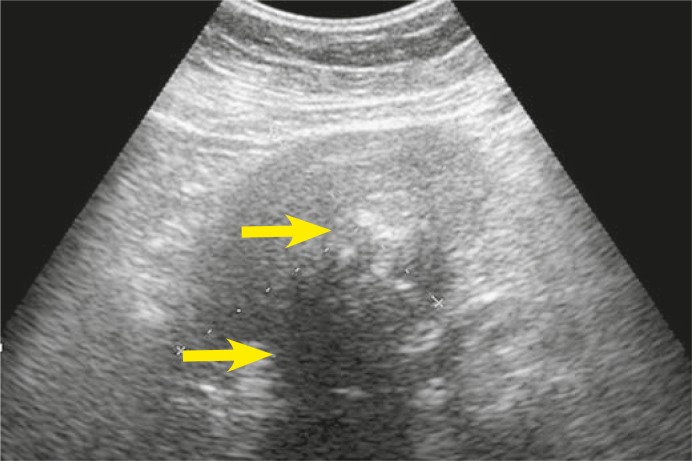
The sonographic appearance of an intra-abdominal desmoid with involvement of the small bowel: a well-defined grossly homogenous hypoechoic mass circumferentially encroaching the intestinal wall; the hyperechoic central part of the tumour corresponds to intraluminal air that results in posterior acoustic shadowing (arrows).
